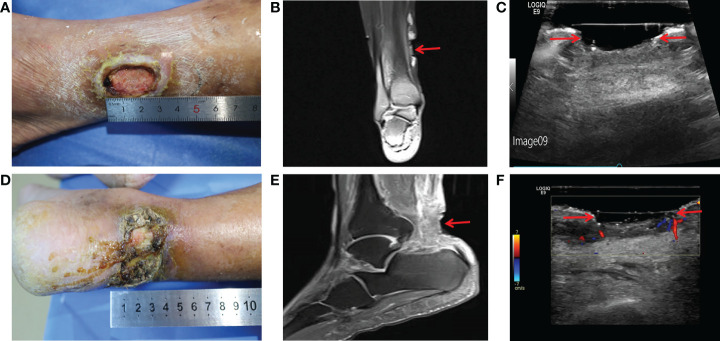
Imaging diagnosis of diabetic lower extremity ulcers before treatment for case 1 (A-C) and case 2 (D-F). (A) Picture showed an ulcerated surface on the inner skin of the right calf, about 3.0×2.0 cm in size and 6 mm in depth; (B) MRI showed a local subcutaneous soft tissue defect at the medial margin of the right calf, with swelling in the margin and adjacent soft tissue space; (C) Gray-scale sonography showed a heterogeneous low echo area in the subcutaneous soft tissue of the medial side of the right calf; (D) Picture showed an ulcerated surface in the skin of the right heel, about 7.5 × 4.6 cm in size and 4 mm in depth, with necrosis and exudation; (E) MRI showed extensive swelling of the soft tissue and fascia in the lower part of the right calf with unclear and disordered layers; (F) CDFI showed local skin defects and discontinuity in the skin of the right heel. The blood flow signal in the low echo surface was not obvious, and a little blood flow signal could be seen in the periphery. The red arrows indicate the ulcer defects.
